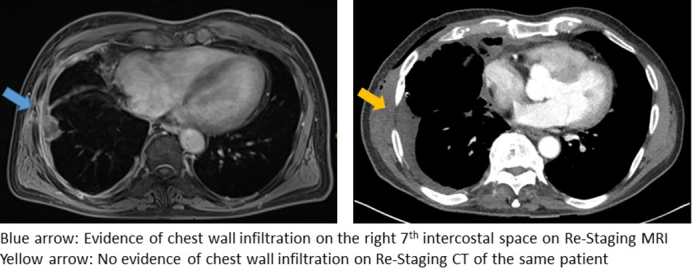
Example of better detection of mesothelioma thoracic wall infiltration on MRI.
